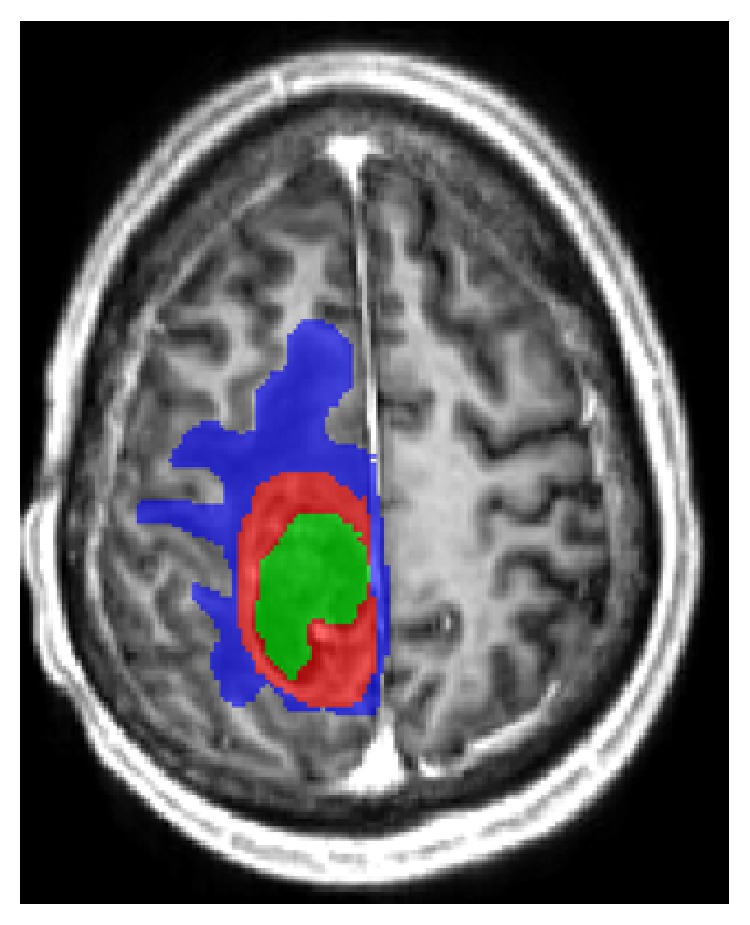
Delineations on T1 MR image postcontrast. Green—necrosis, red—CE, and blue—ED.
